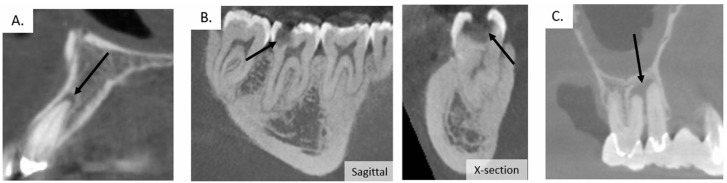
Cone beam computed tomography (CBCT) images detect the presence of apical inflammatory lesions. This type of empirical evidence for oral infections is used as a method of deciding treatment course by dentists. (A) CBCT sagittal image shows an apical inflammatory lesion as a darker grey pocket surrounding the apex of tooth. (B) CBCT images show dental caries from sagittal and cross-sectional views. (C) Sagittal CBCT image shows large apical inflammatory lesion between the apices of adjacent teeth. From the author’s collection.
